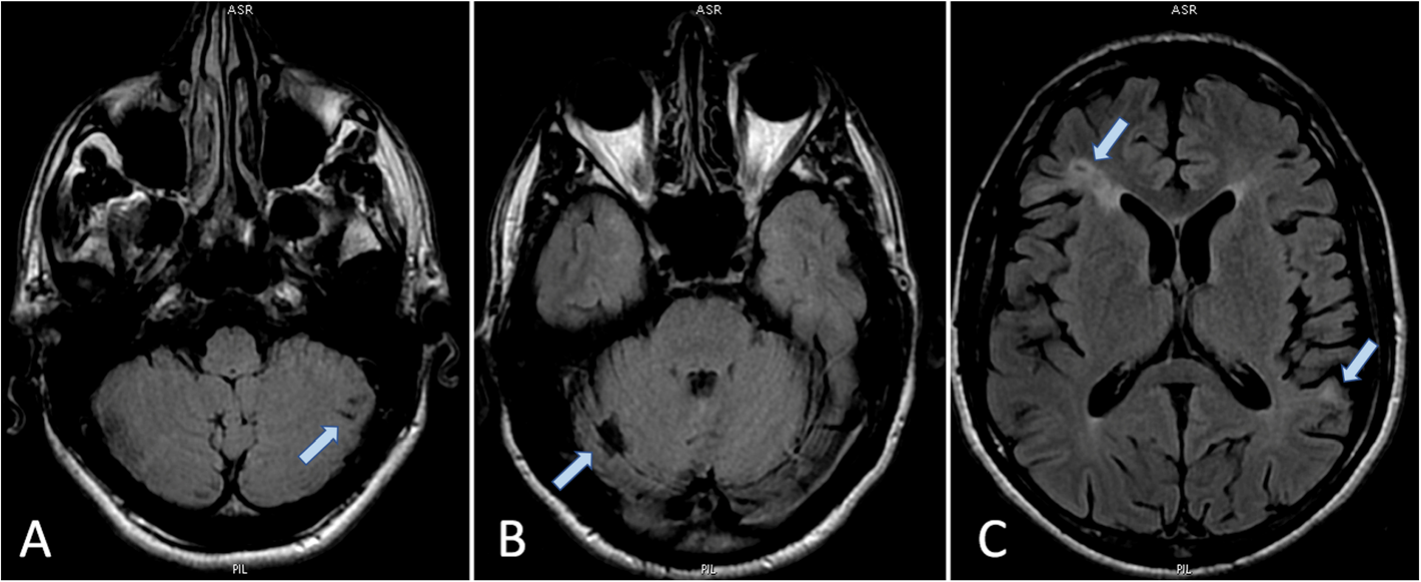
Brain T2-FLAIR MRI scans showing multiple areas of ischemia (*light blue arrows*) in the cerebellar and cerebral hemispheres. a Recent ischemic lesions in the lower left cerebellar hemisphere. b Old ischemic lesion in the upper right cerebellar hemisphere. c Recent ischemic lesions in the right frontal and left temporo-occipital cerebral regions
